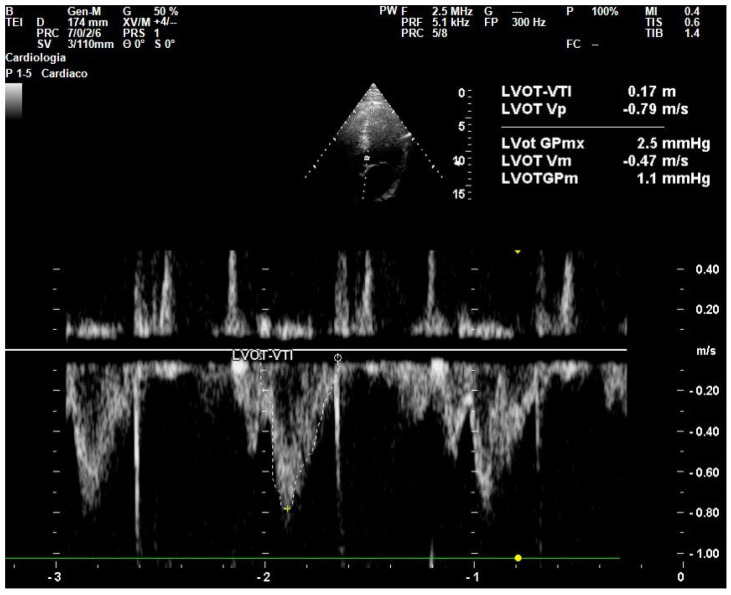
Example of Doppler with pulsed waves in the LVOT and measurement of the VTI tracing in apical 5-chamber echocardiographic view.
